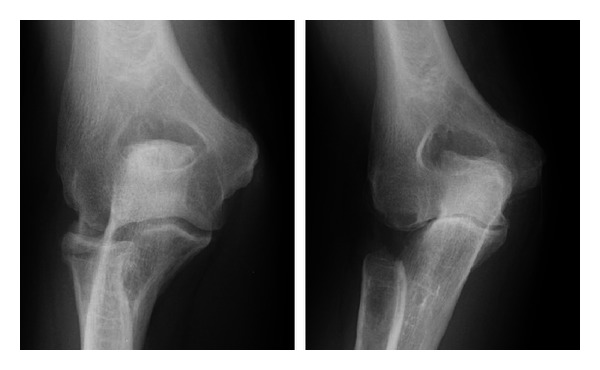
A young male laborer with symptomatic arthrosis of the radiocapitellar joint (A) underwent radial head resection; follow-up radiographs demonstrate progressive ulnohumeral arthritis approximately 18 months following the surgical procedure.
